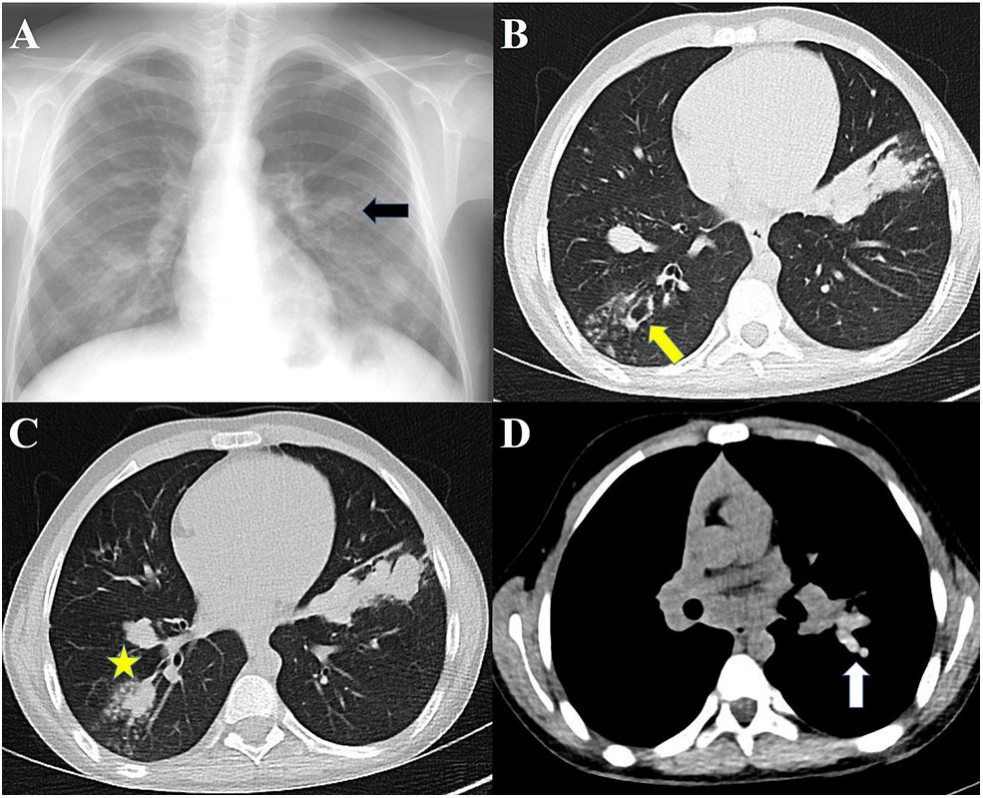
Radiographs of patients with ABPA. (A) Chest radiograph showing finger-in-glove sign, (B) HRCT showing central bronchiectasis, (C) HRCT showing mucus plugging in dilated bronchi, (D) HRCT showing high-attenuation mucus.
